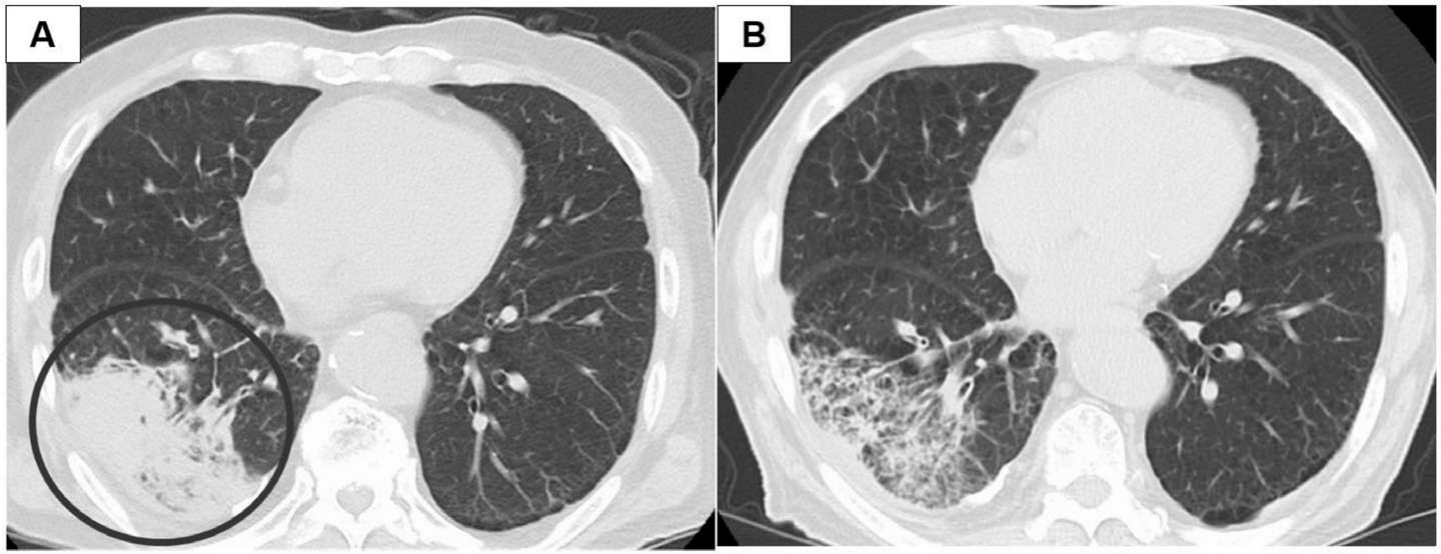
(A) Computed tomography showed a primary tumor in the S9 of the right lung (circle). (B) Computed tomography showed that the tumor had shrunk and its attenuation had decreased.
